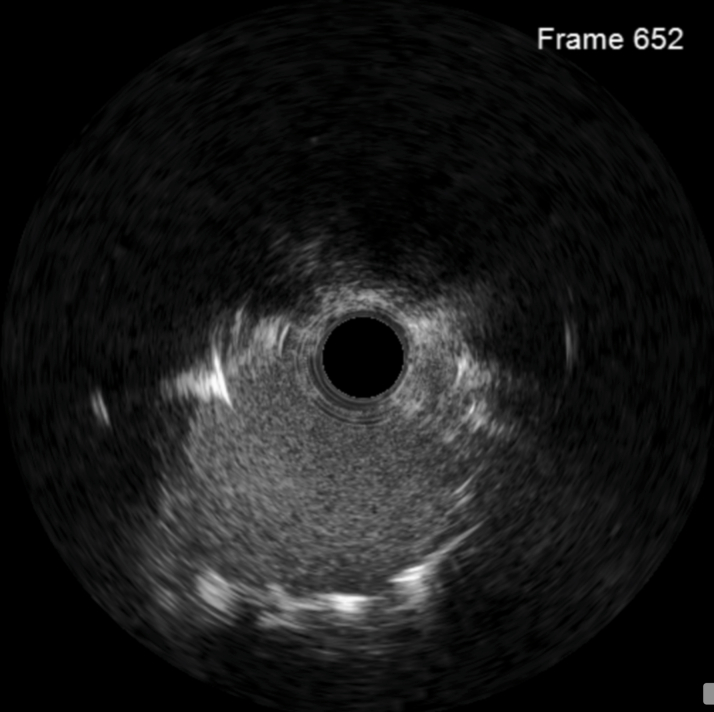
Final Intravascular Ultrasound Following Stent Placement at Location of Minimal Stent Area Just Outside Aorta, Which Was 9.1 mm^2^
